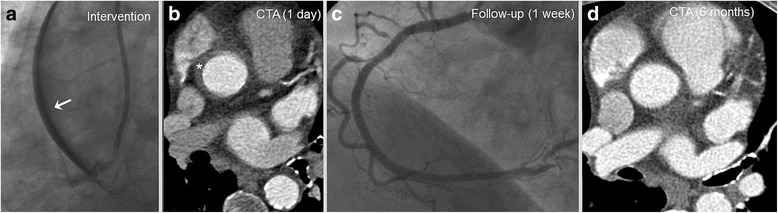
Dunning Dissection type III. Patient presenting with unstable angina in the chest pain unit. a Complex intubation of the RCA ostium with AR2 results in a Dunning Typ 3 dissection (arrow) with emergency sealing (Graftmaster 3.5/16 mm) without further progress of the dissection. b One day after the initial intervention CT confirmed an extensive dissection. c The invasive follow-up 9 days later showed good result, with subsequent successful re-intervention of the RCA and the LMT. d The follow-up cCTA confirms complete recovery after a follow-up period of 6 months. cCTA, coronary CT angiography; RCA; right coronary artery
